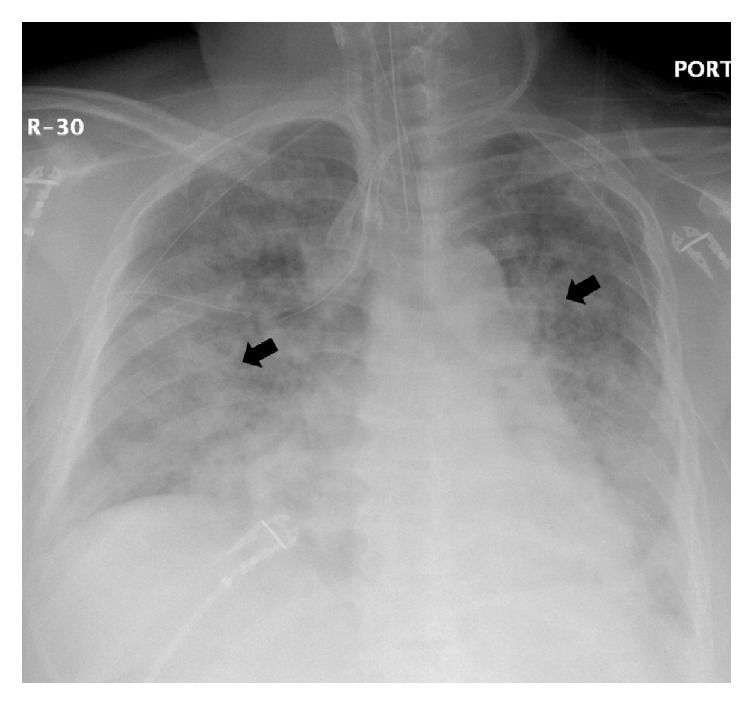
Respiratory failure on postoperative day 2. Shown is the chest X-ray with bilateral patchy opacities (black arrows) after urgent reintubation. Together with a normal left ventricular function and poor oxygenation (PaO_2_ 60 mmHg, FiO_2_ 0.8, Horowitz quotient of 75), an acute respiratory distress syndrome (ARDS) was diagnosed, based on the Berlin definition.
